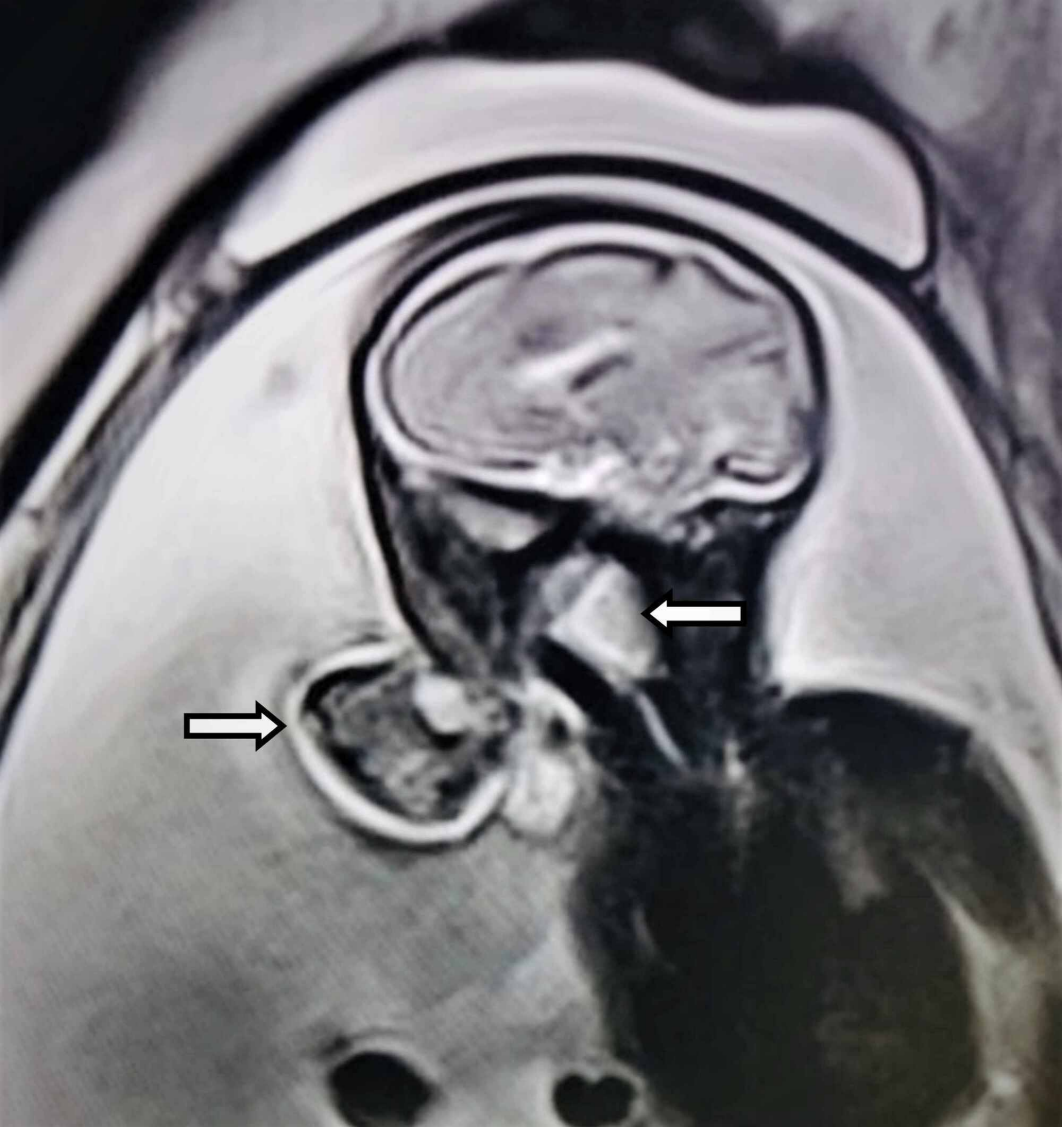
Sagittal MRI scan showing a mixed intensity mass with a stalk seen protruding through jaws into the amniotic fluid. The internal portion of mass emerging in relation to the hard palate and filling the oropharynx. There is no associated brain anomaly or intracranial mass invasion. MRI, magnetic resonance imaging.
